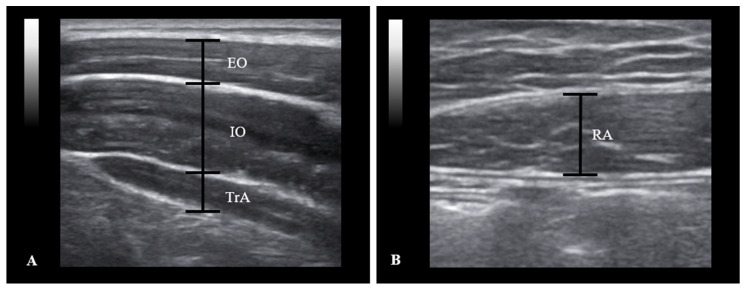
Abdominal muscle ultrasound image obtained in the right horizontal position. (A) TrA: transverse abdominis; IO: internal oblique; EO: external oblique; (B) RA: rectus abdominis.
